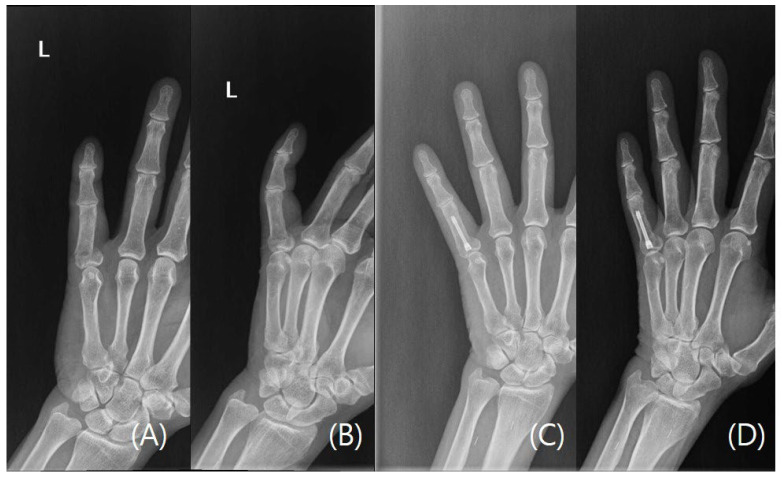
A case of a left fifth digit transverse proximal phalangeal fracture. (A) Preoperative plain radiograph Posteroanterior view. (B) Preoperative plain radiograph oblique view. (C) Postoperative plain radiograph Posteroanterior view taken at last follow-up visit. (D) Postoperative plain radiograph oblique view taken at last follow-up visit. The letter L refers to the left hand.
